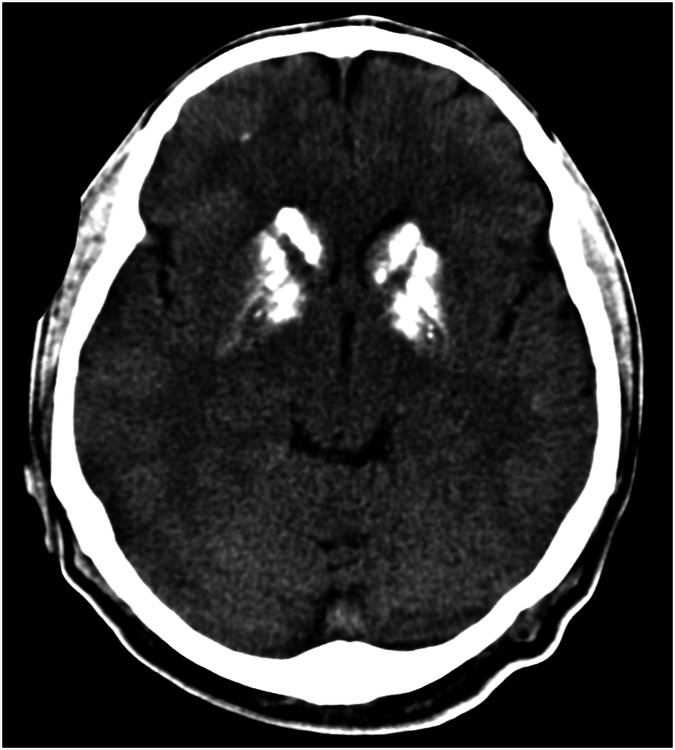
Fahr disease. CT image displays calcifications in regions rich in ferritin
